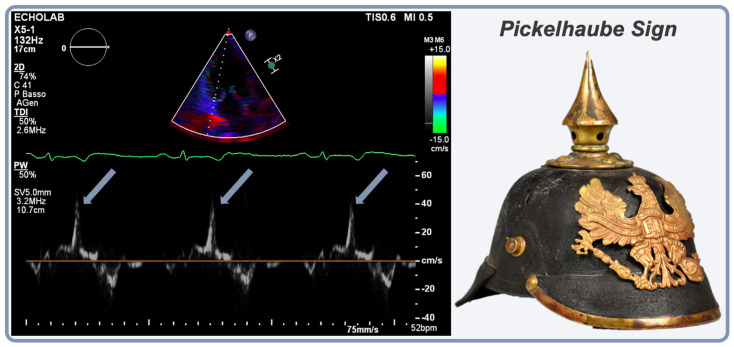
Pickelhaube sign, a distinctive spike during systole of the lateral mitral annulus using tissue Doppler.
